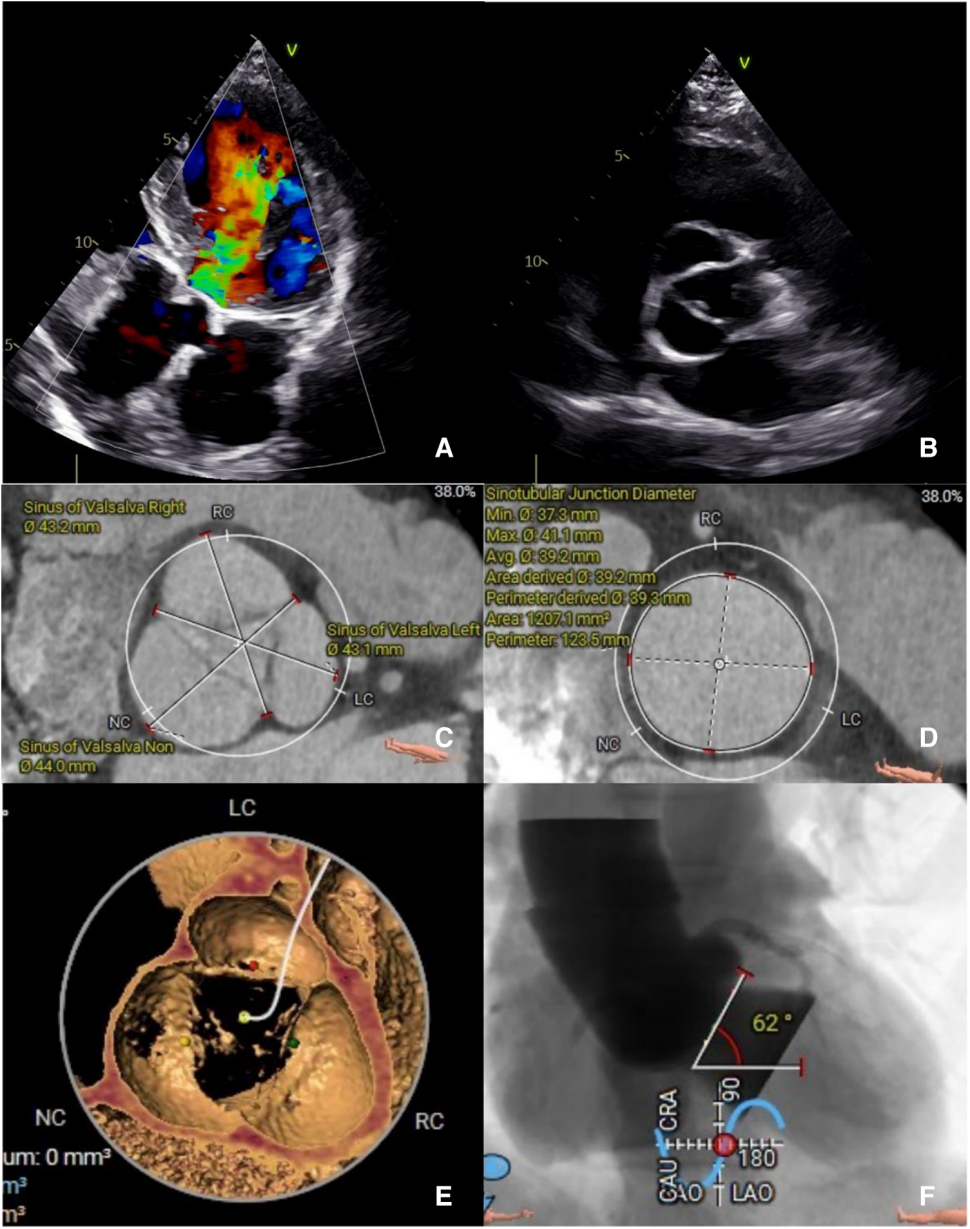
(A) The preoperative transthoracic echocardiogram in diastole showing severe AR and (B) absence of annulus and leaflets calcification. A preoperative computed tomography evaluation (C,D) showing a large sinus of Valsalva and sinus tubular junction. (E) No annulus of leaflets calcification. (F) Aortic angulation.
